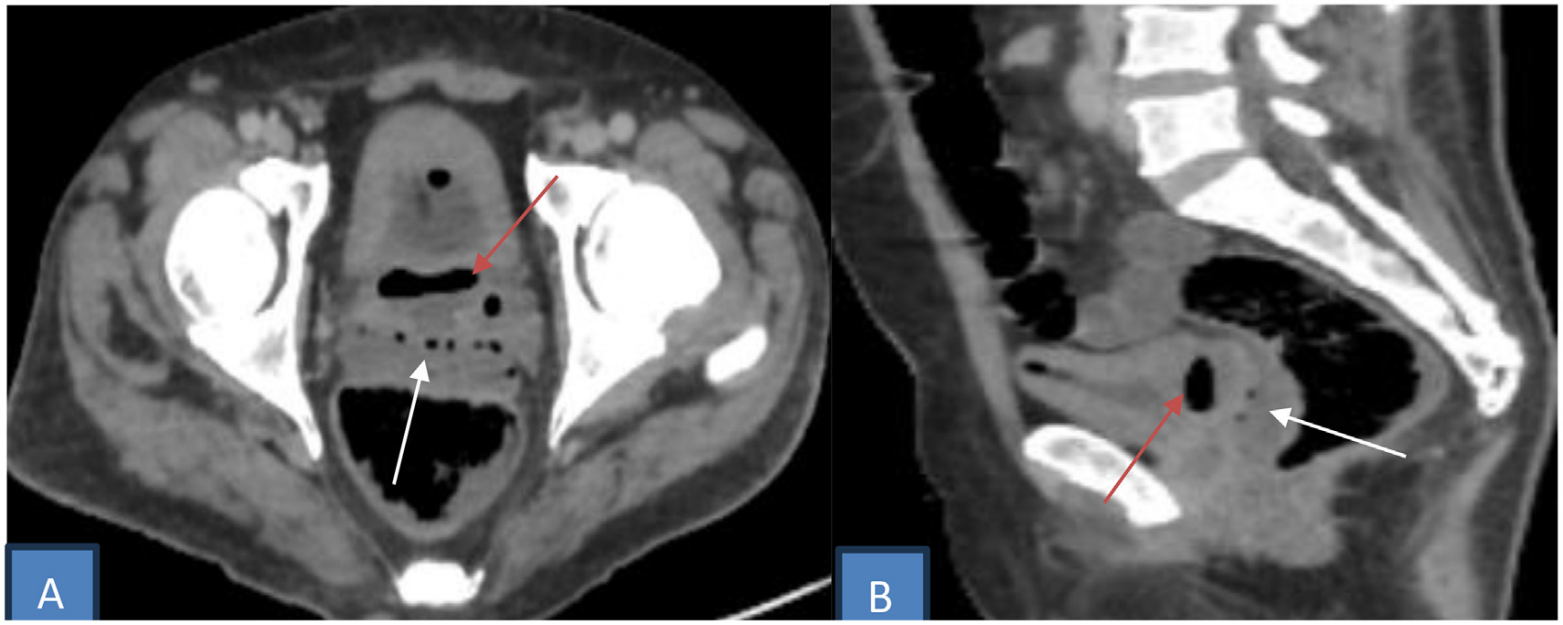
Axial (A) and sagittal (B) pelvic CT image showing marked thickening of both the anterior and posterior bladder walls. Gas bubble adherent to the anterior wall, with another in the posterior bladder wall (red arrow), consistent with emphysematous cystitis. A pelvic abscess containing air in the rectovesical pouch (white arrow) suggesting bladder rupture.
